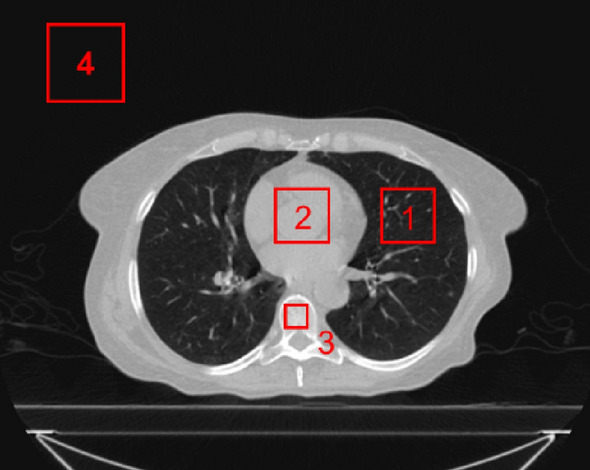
Position (1 to 4) of each ROI for calculating the mean error and mean absolute error compared with the original CT image. ROI (1 to 4) positions of the lung, soft tissue, bone, and air regions, respectively.
